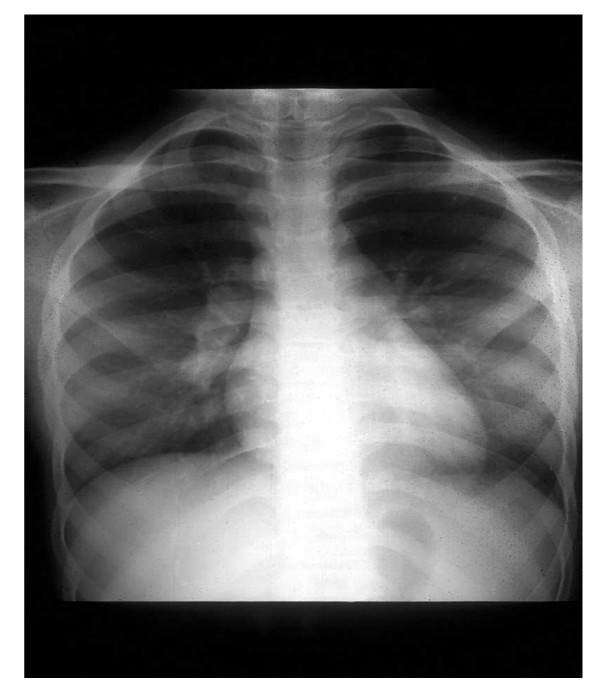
Chest radiograph in a 10-year-old female with sarcoidosis showing hilar adenopathy.
